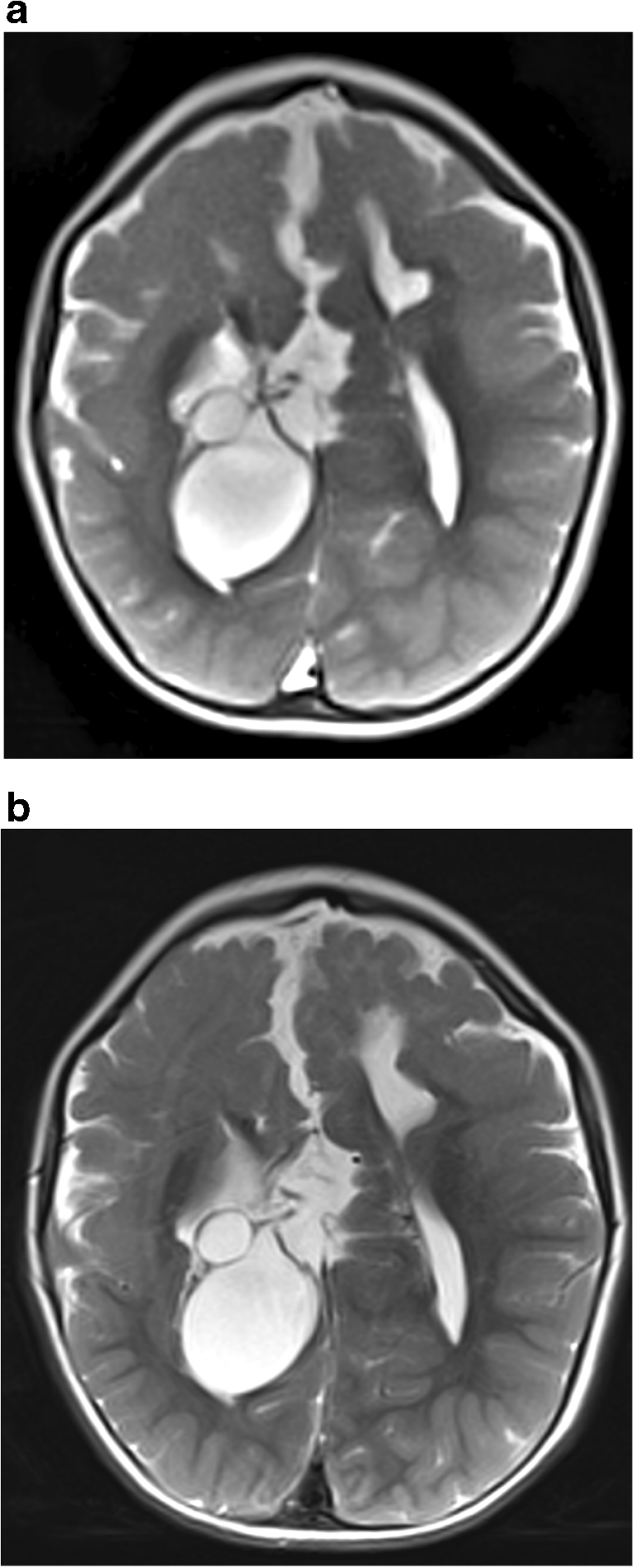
Comparison of axial image quality at rest in a 5-year-old girl with Aicardi syndrome, callosal agenesis and interhemispheric cysts. a Volume coverage scan with refocused fast low-angle shot contrast (T2/T1). b Conventional T2-weighted fast spin echo
